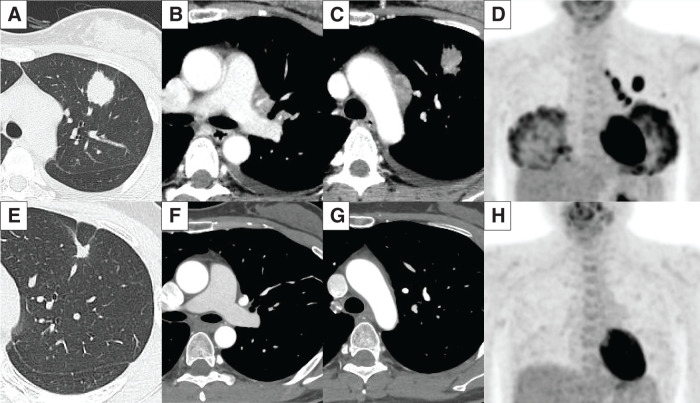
(A–D) Pretreatment clinical stage was Stage IIIA (cT1cN2M0) according to the 8th edition of UICC/IASLC TNM classification. (A) Contrast-enhanced chest CT scan showed a 25-mm irregular nodule in the left upper lobe segment 3. (B) Enlarged left hilar lymph nodes at stations 12u and 13 were present, suggesting possible invasion of A^3^ and the left pulmonary artery. (C) Enlarged mediastinal nodes at stations 5 and 6. (D) Fluorine-18-fluorodeoxyglucose PET-CT showed intense metabolic activity in both the primary lesion (the maximum standardized uptake value rising from 11.3 to 15.0) and the involved lymph nodes, with no evidence of distant metastasis. (E–H) After 11 months (342 days) of treatment, corresponding to restaging as Stage IA2 (ycT1bN0M0). (E) The primary tumor had shrunk by 55.3%—from 25 to 11 mm. (F, G) The previously enlarged nodes regressed. (H) There was complete resolution of fluorine-18-fluorodeoxyglucose uptake. A^3^, the segment 3 arterial branch; IASLC, International Association for the Study of Lung Cancer; UICC, Union for International Cancer Control
